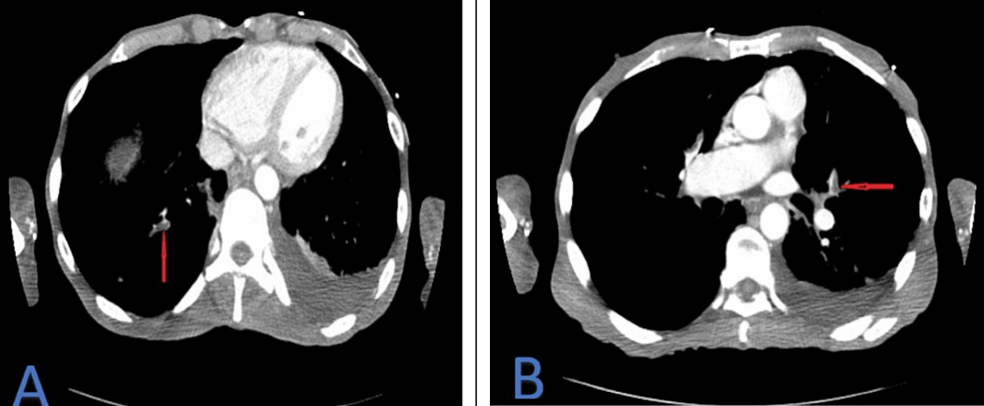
Computed tomography (CT) of the chest with contrast in the axial sections (A and B) with red arrows pointing towards filling defects in the subsegmental arteries in the bilateral lower lobes
